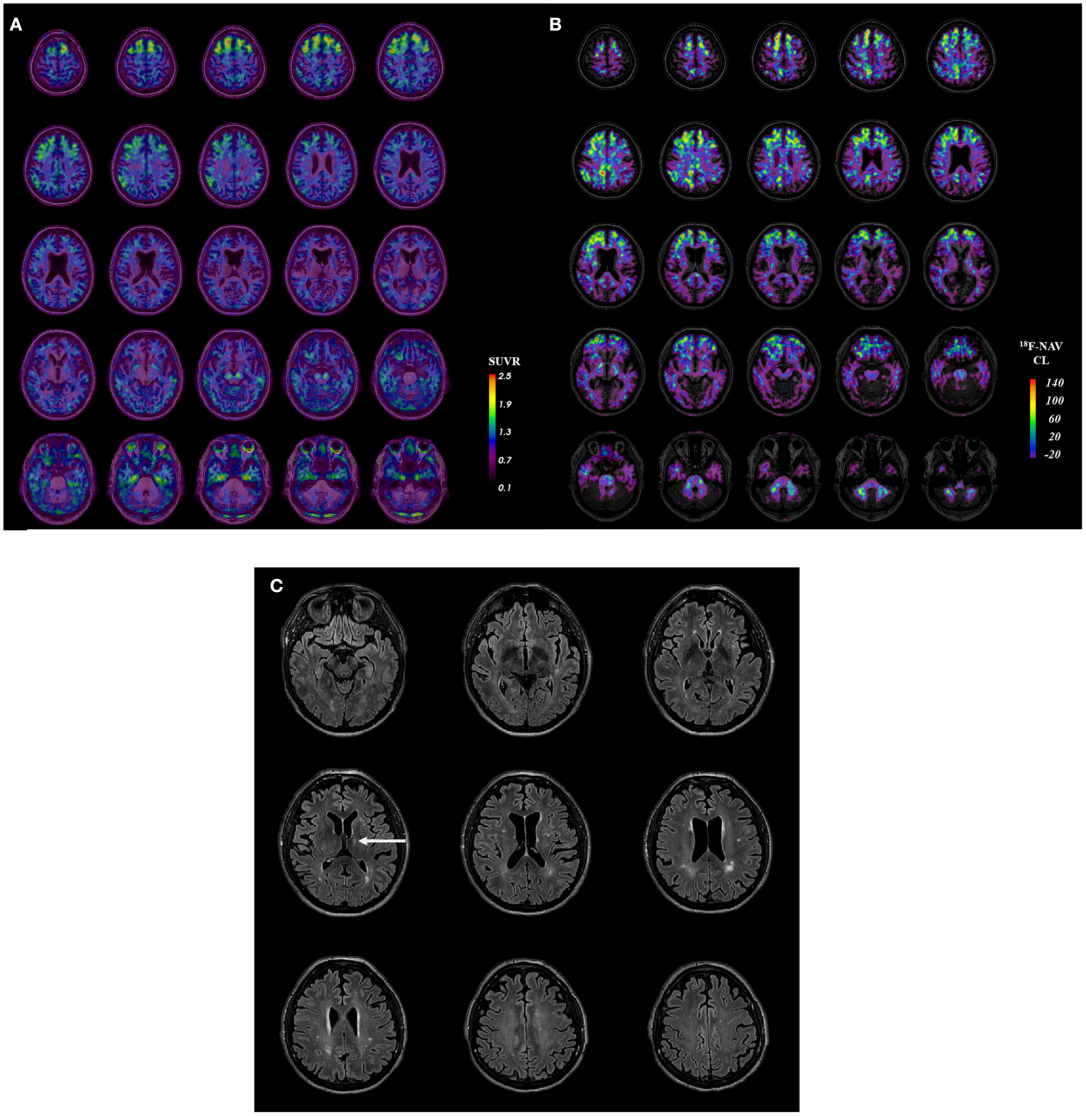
Case participant's. (A) Tau PET image. (B) Amyloid PET image. (C) Axial FLAIR image. Participant (A)
